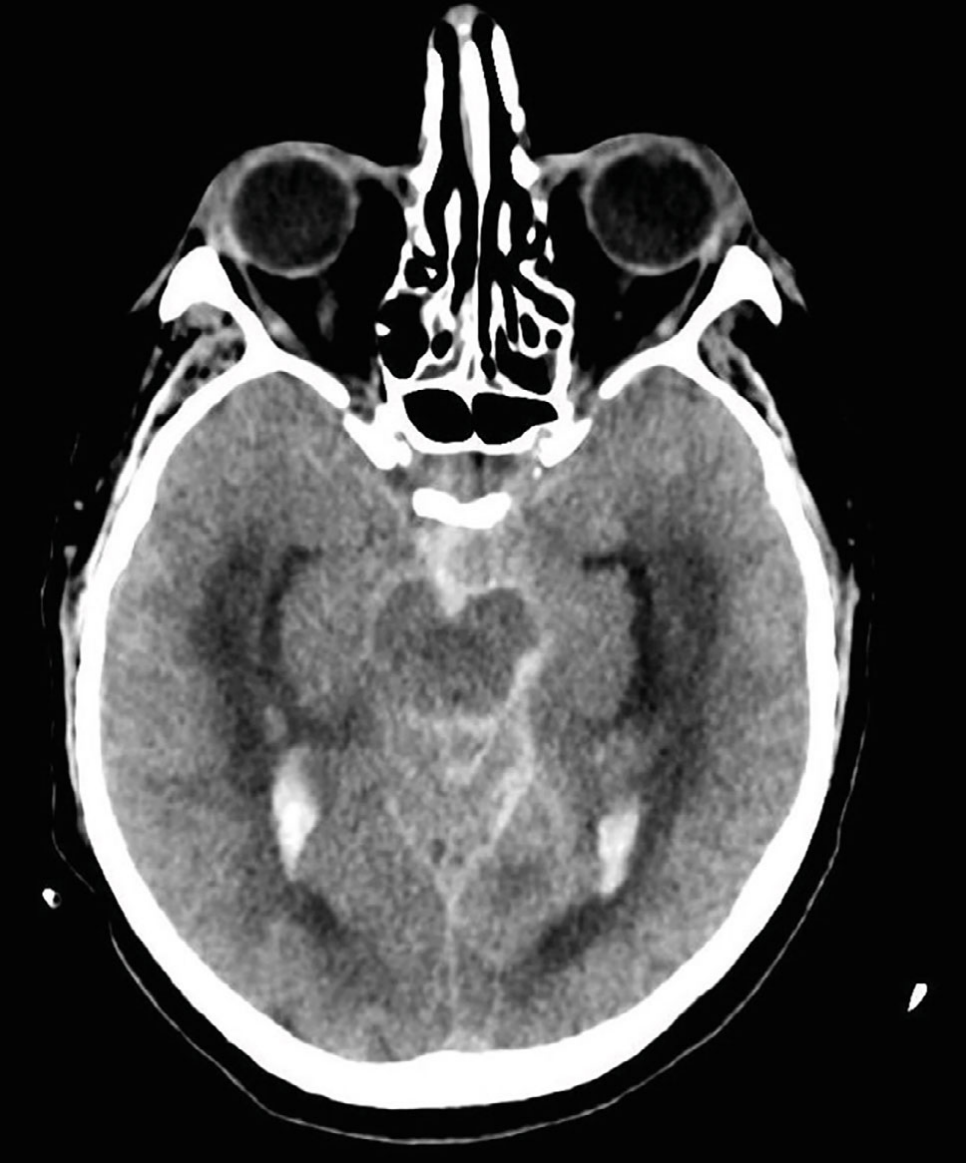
Head CT scan on admission.
